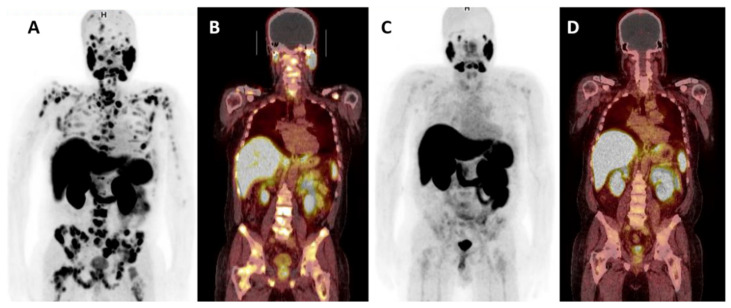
^177^Lu-PSMA-617 treatment results in metastatic castration-resistant prostate cancer. ^68^Ga-PSMA PET maximum intensity projection (MIP) images, (A,C) and coronal PET-CT (B,D) whole-body images before (A,B) and after (C,D) four cycles of ^177^Lu-PSMA-617 show almost complete disappearance of PSMA-positive metastatic tumors after radionuclide treatment in a patient with metastatic castration-resistant prostate cancer (mCRPC). Image: J. Kemppainen, Docrates Cancer Center.
